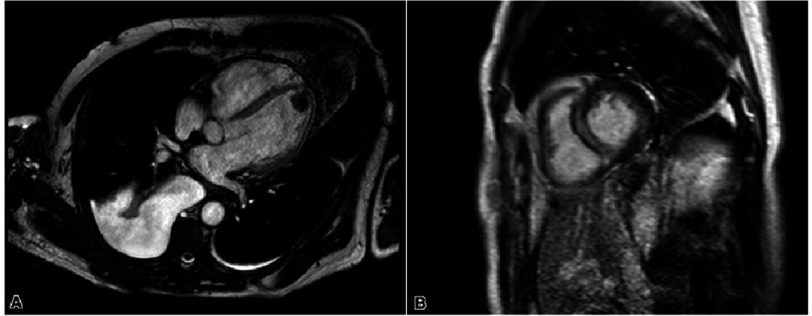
Four-chamber (A) and short-axis (B) cardiac magnetic resonance imaging demonstrating apical thrombus and focal delayed mid-wall myocardial enhancement at the basal portion of the interventricular septum consistent with myocarditis versus infiltrative process.
